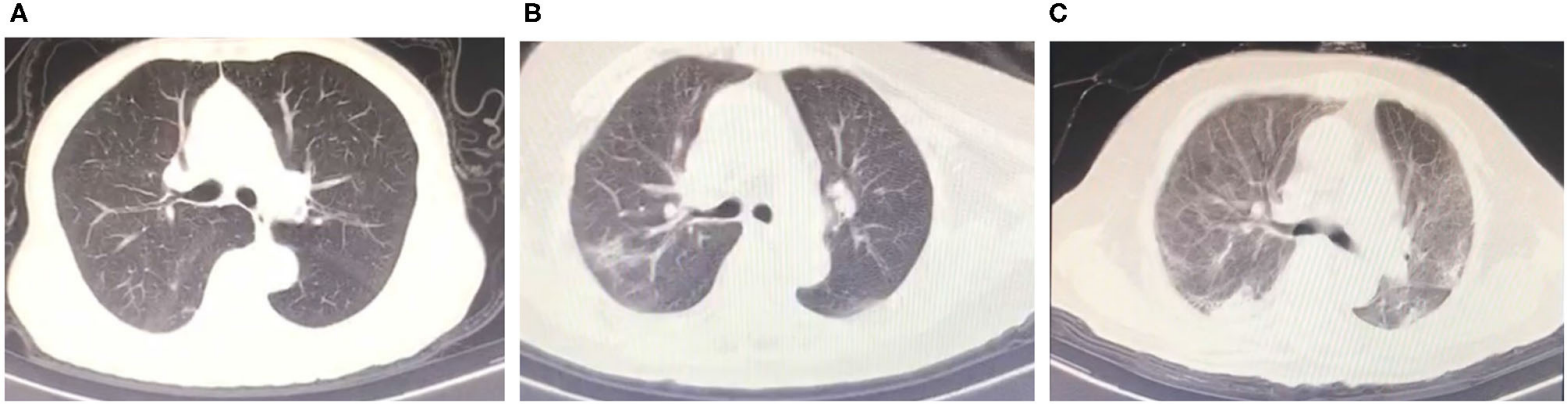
Chest CT scans and CEA levels of three female patients. (A) Four points CT scores and 0.9 ng/ml CEA levels. (70 years old). (B) Sixteen points CT scores and 3.1 ng/ml CEA levels (70 years old). (C) Twenty two points CT scores and 7.7 ng/ml CEA levels (69 years old).
